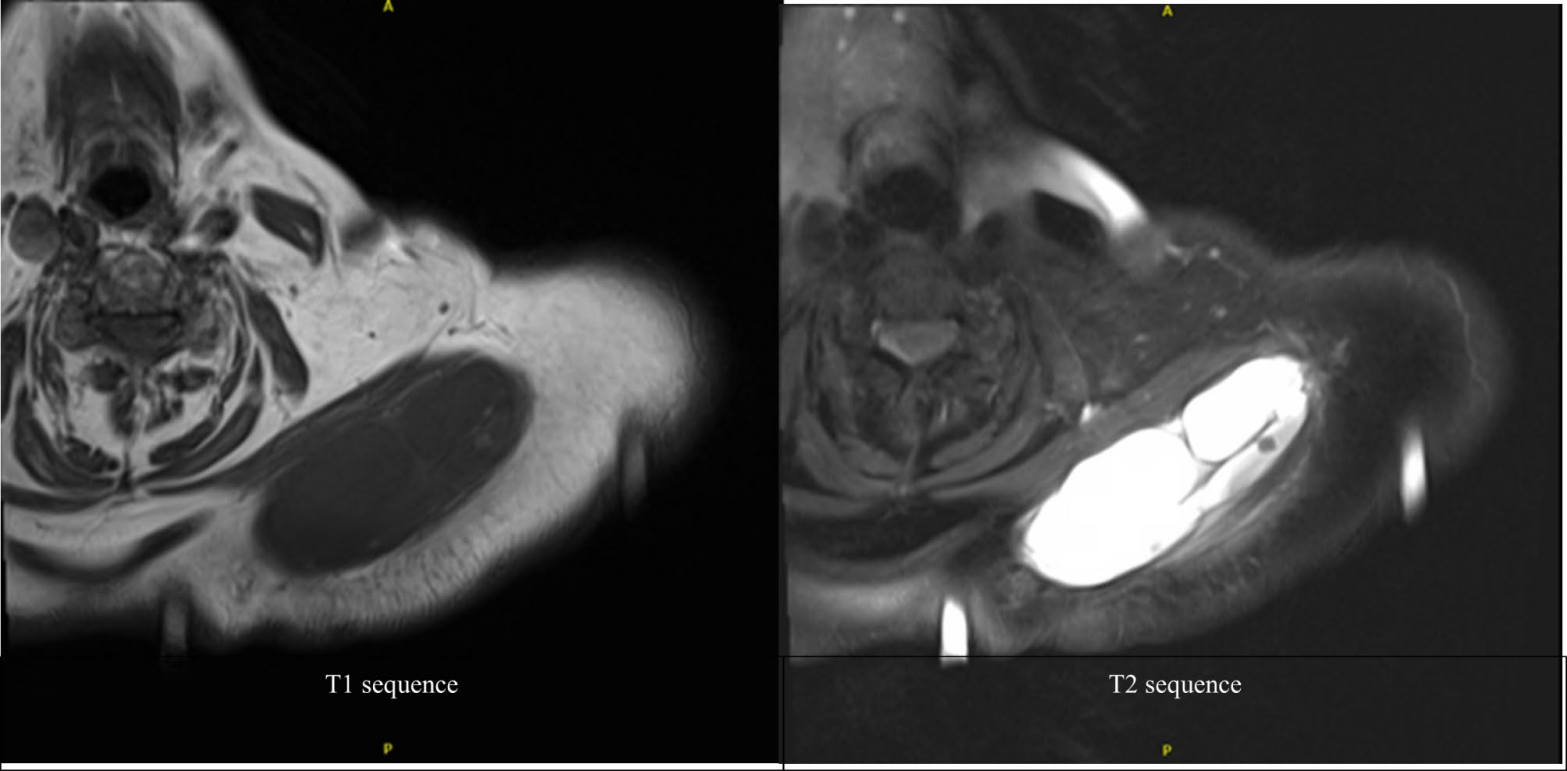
MRI of the cyst demonstrating 10.5 cm x 3.4 cm x 4.4 cm mass originating from the AC joint with peripheral and septal enhancement on T1 and T2 axial sequences
